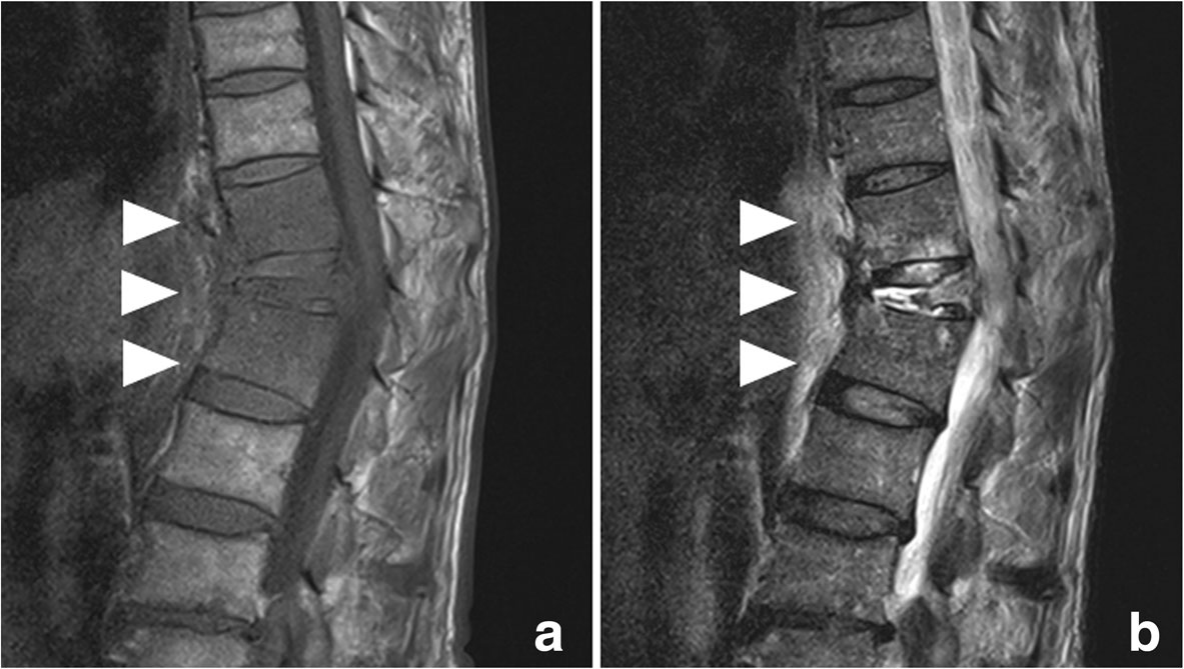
Postoperative lumbar MRI. a, b MRI revealed low T1-weighted signals and high T2-weighted signals in the 12th thoracic vertebral body, and the signals spread to the upper and lower intervertebral disk space (white arrowheads)
